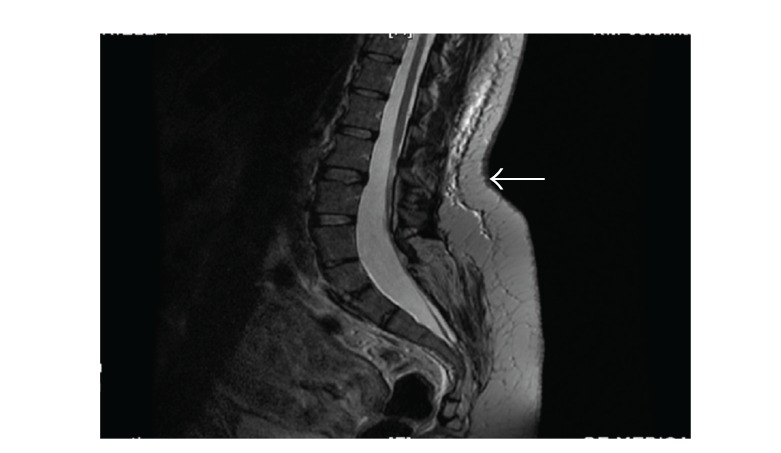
Sagittal view of the patient's MRI scan with spinal cord. The arrow approximately indicates puncture site.
